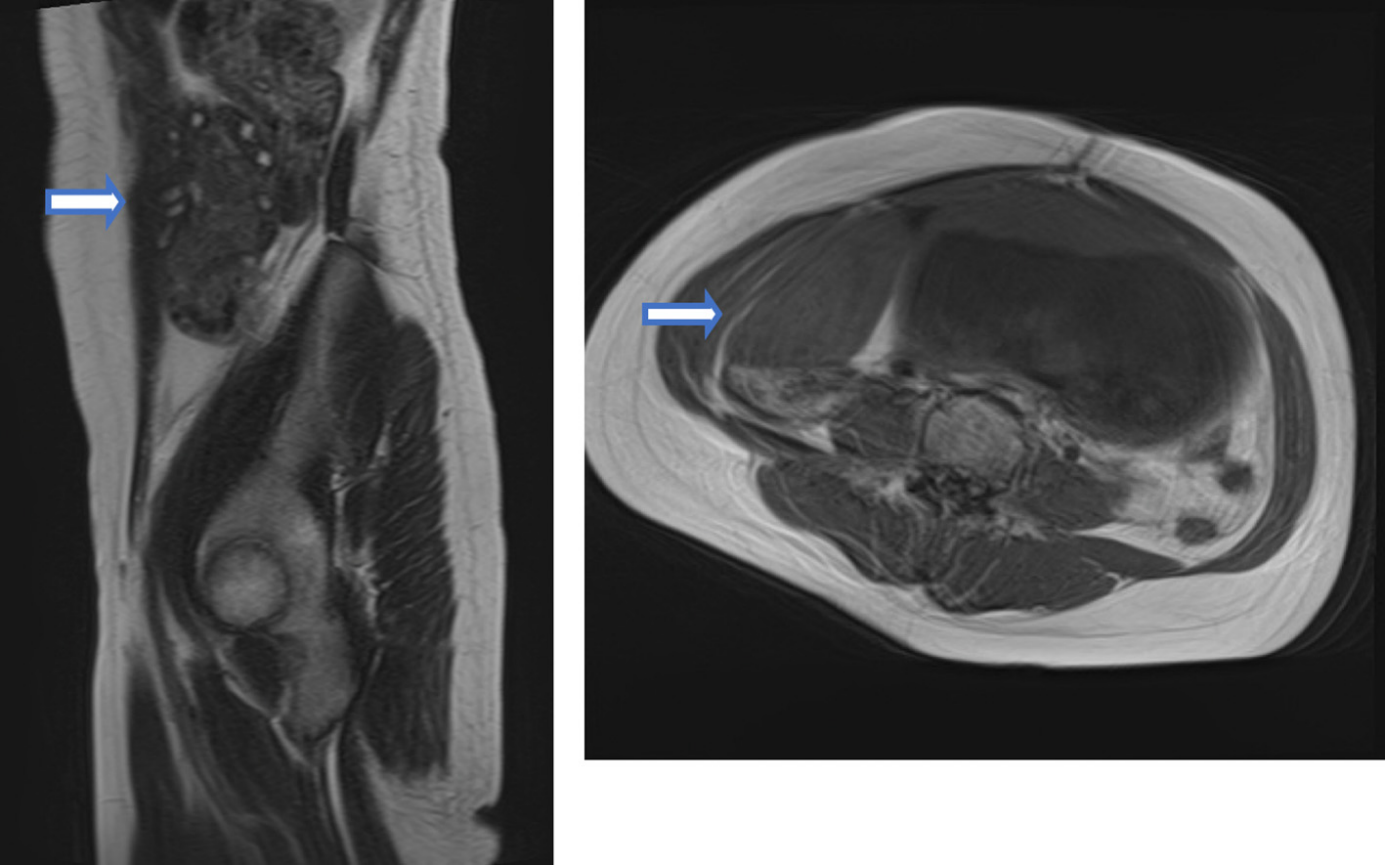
Magnetic resonance imaging (Left; T2-weighted image/sagittal, Right; T1-weighted image/axial). Multiple ovarian follicles were noted in the periphery of the mass on the T2-weighted image.
